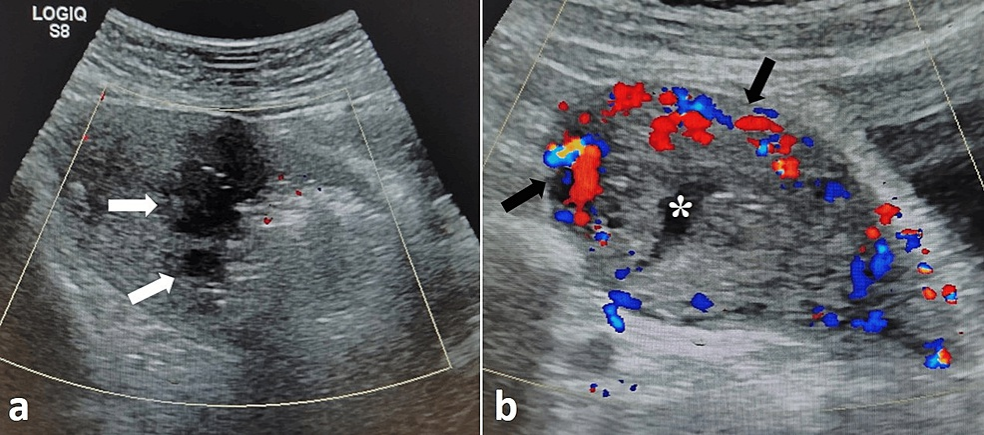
US images of the patient with AVM Post-UAE: (a) Transabdominal US image on day 1 of intervention showing bulky uterus with few variable-sized anechoic cystic structures within the endometrial cavity (white arrows); however, no abnormal vascularity was detected in the uterine wall on Color Doppler. (b) Transabdominal US image after three months showing a normal-sized uterus with normal uterine vessels (black arrows) and minimal endometrial collection (asterisk). AVM, arteriovenous malformation; UAE, uterine artery embolization; US, ultrasound
